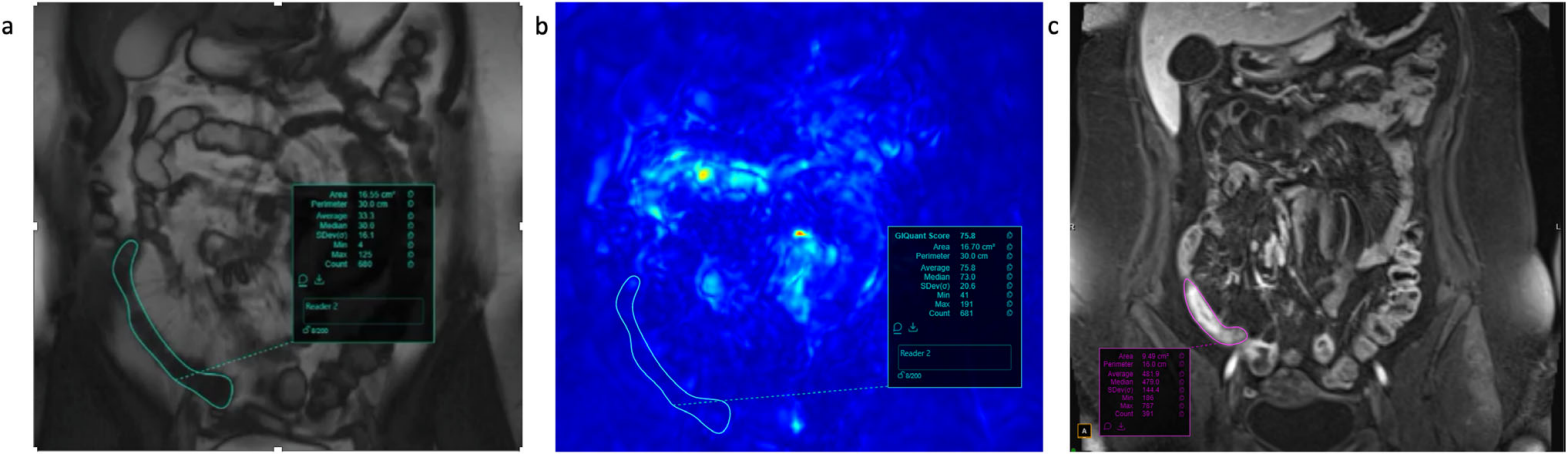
Motility MRI in a patient with active terminal ileitis due to CD. (a) Reference balanced steady‐state free precession anatomical image with ROI in the area of involvement in the TI; (b) motility MRI parametric map. Red depicts areas of high motility while blue areas of low motility. The motility map indicates reduced motility in the affected region. The GIQuant (intestinal motility) score was 75.8; (c) T1 fat‐saturated postcontrast image for reference, showing enhancement of the inflamed TI. CD = Crohn's disease; ROI = region of interest; TI = terminal ileum.
